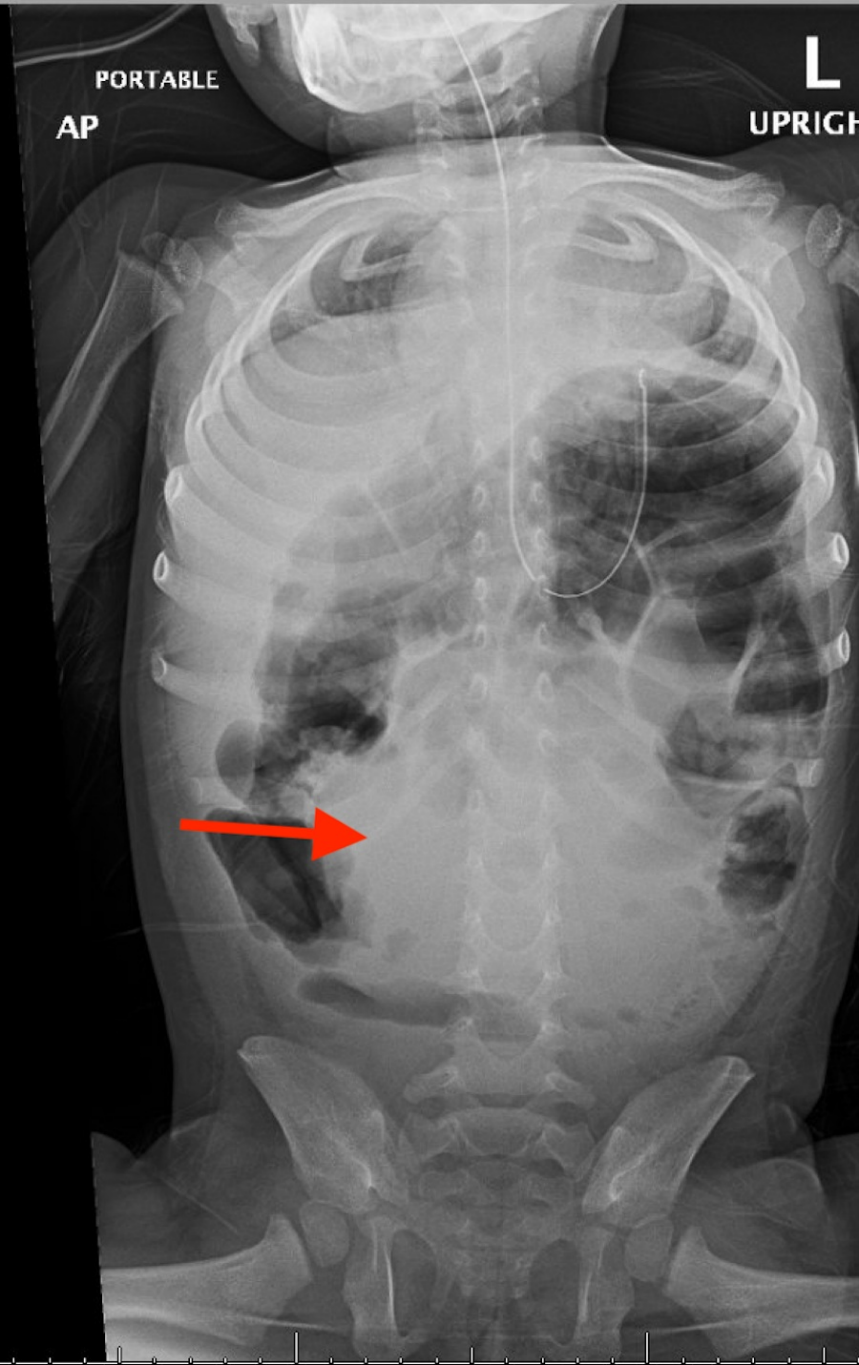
Repeat radiograph on day two of admission, demonstrating a midline soft tissue mass that is 9 cm in diameter
